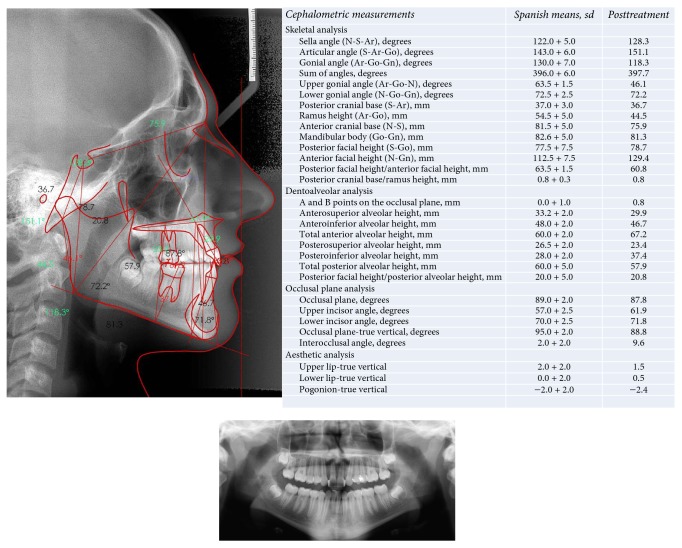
Final panoramic radiograph and lateral radiographic and cephalometric measurements.
